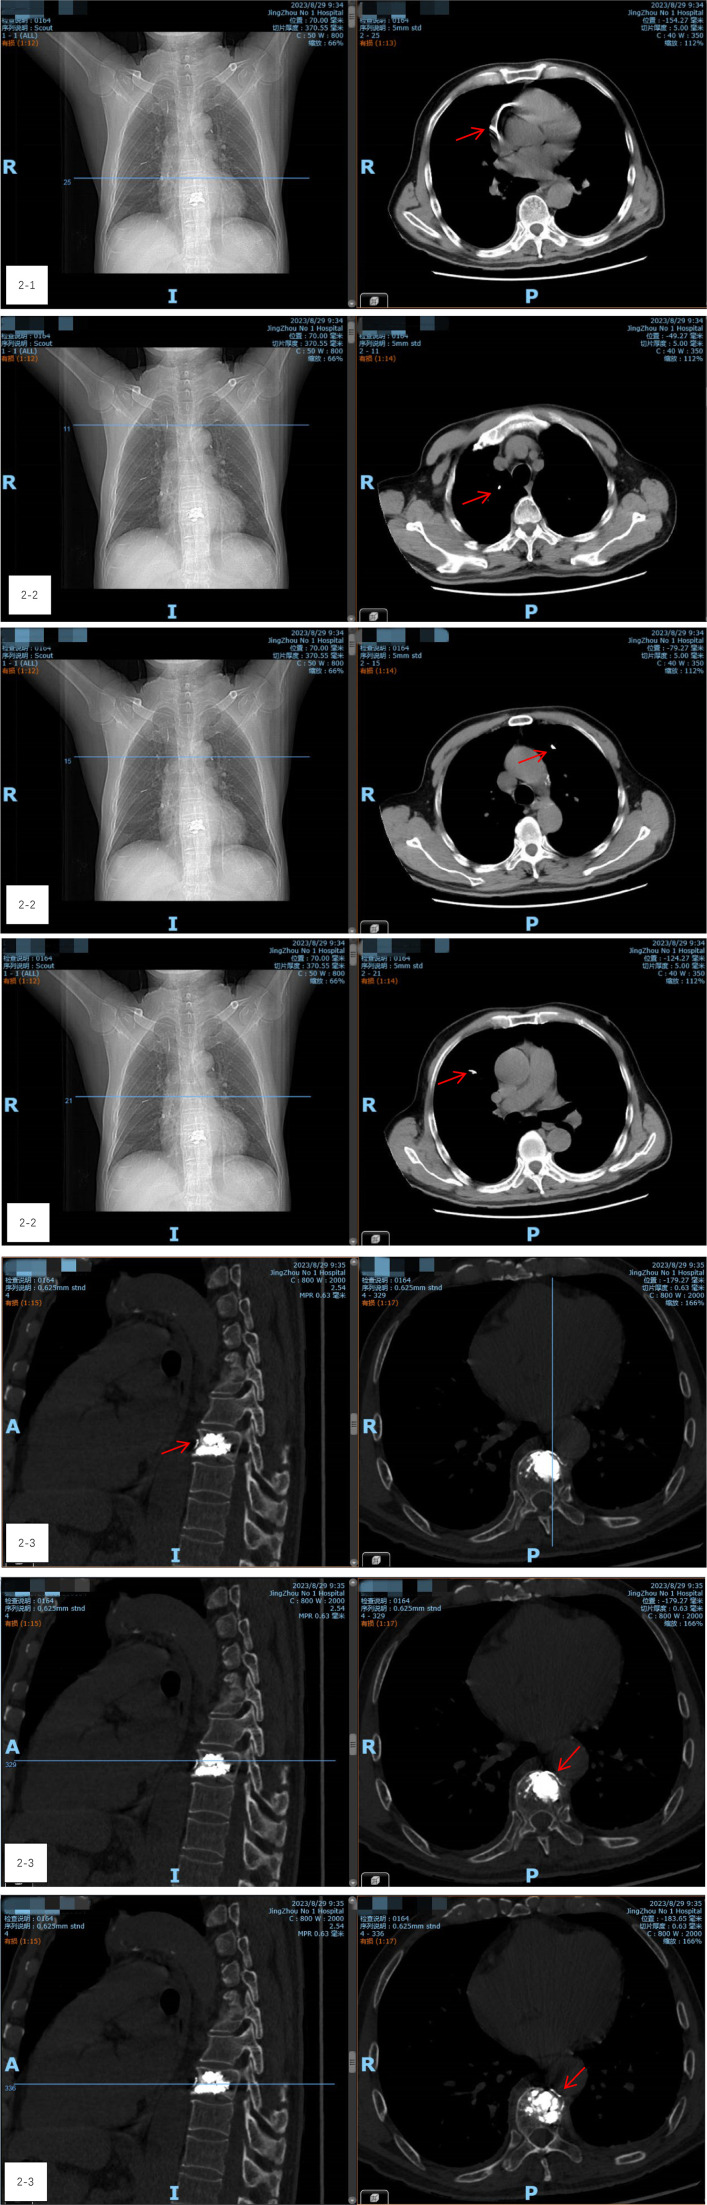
(2-1) A chest CT scan revealed a 5 cm arc-shaped high-density shadow near the right atrium. (2-2) CT shows multiple high-density spots in the lungs. (2-3) A cord-like high-density shadow was detected in the left anterior region of the T9 vertebra.
